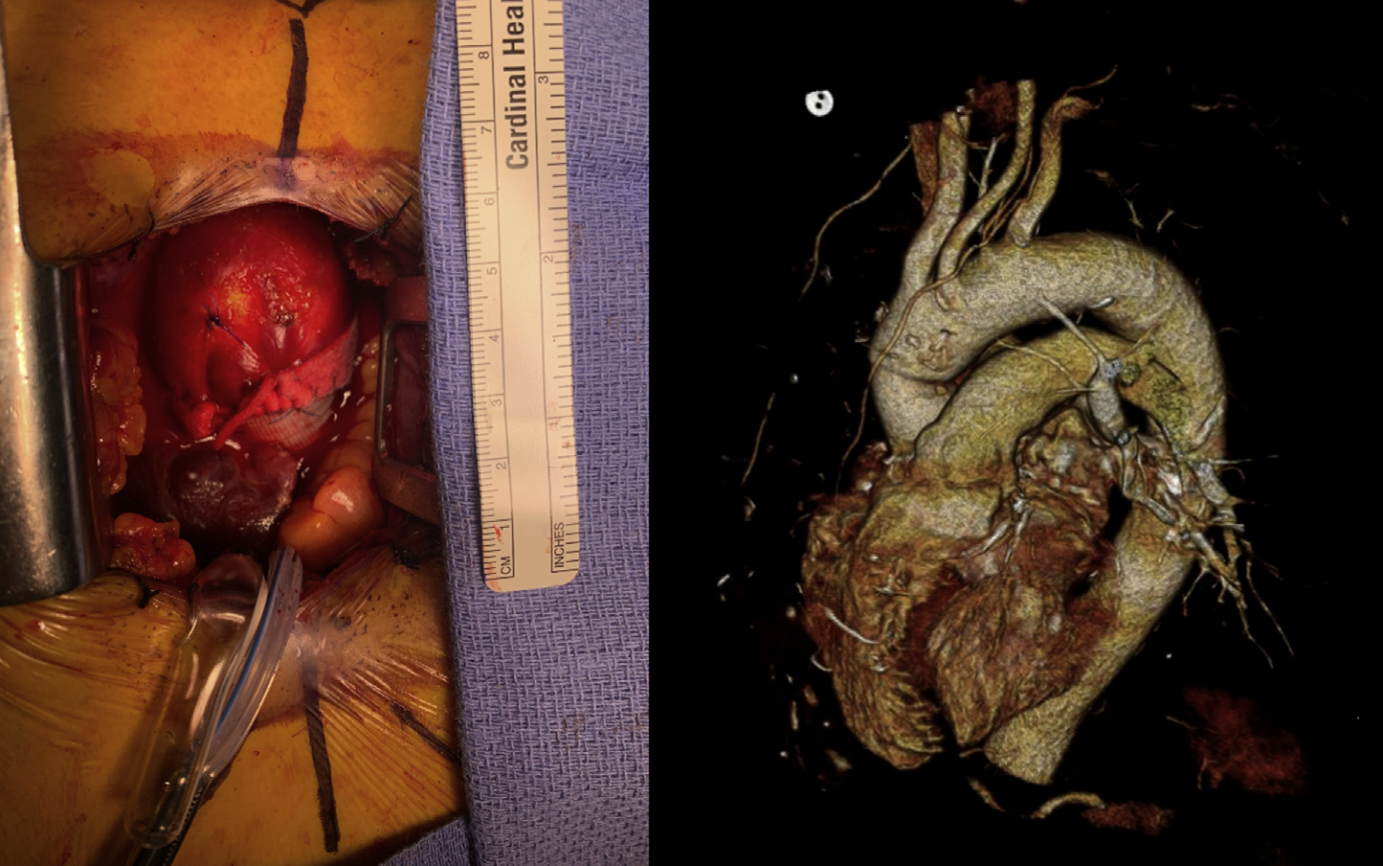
Left*, Intraoperative picture of minimally invasive aortic root and ascending replacement. The mildly dilated distal ascending aorta was left behind. *Right*, Postoperative computed tomography angiogram showing a “size gap” between the graft and mildly dilated distal ascending aorta after an ascending replacement.
